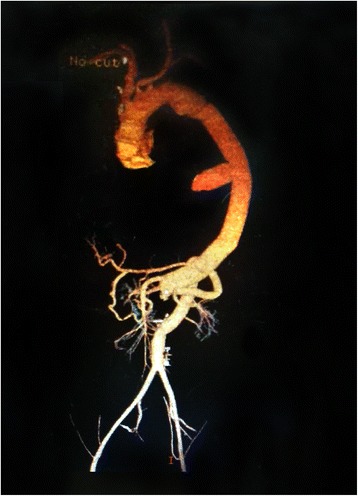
Postoperative computed tomographic scans showed that the thoracoabdominal aortic aneurysm was repaired with a modified multiple branched graft
